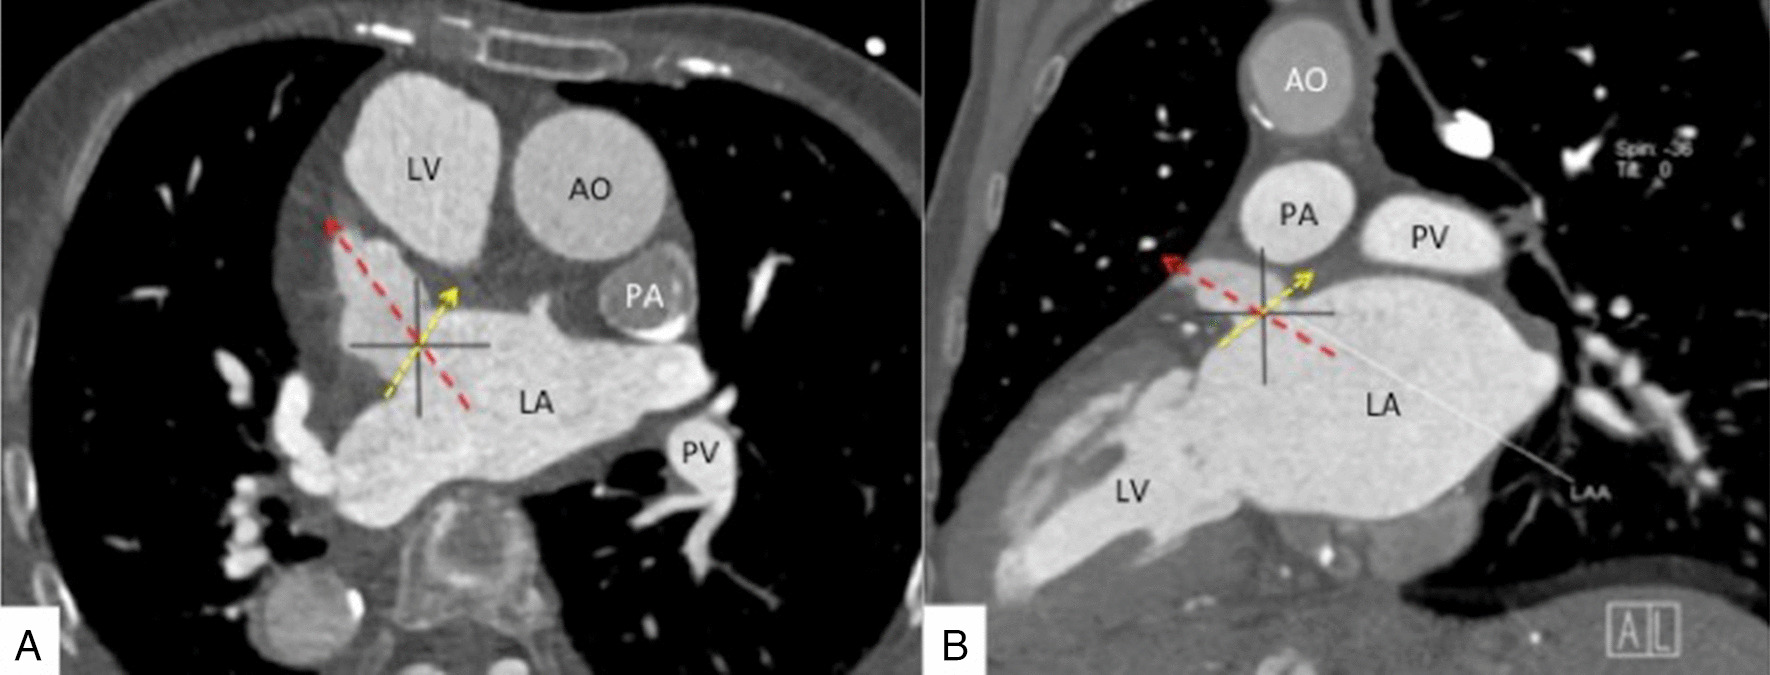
Preoperative computed tomography (CT) guidance. The yellow arrows show the ostium of the left atrial appendage (LAA). The red arrow shows the macro axis of the LAA. The angle of the angiography projection was according to the CT guidance. The best projection angle was LAO 46° + CREA 23°. *AO* aortic, *LA* left atrial, *PA* pulmonary artery, *SVC* superior vena cava
